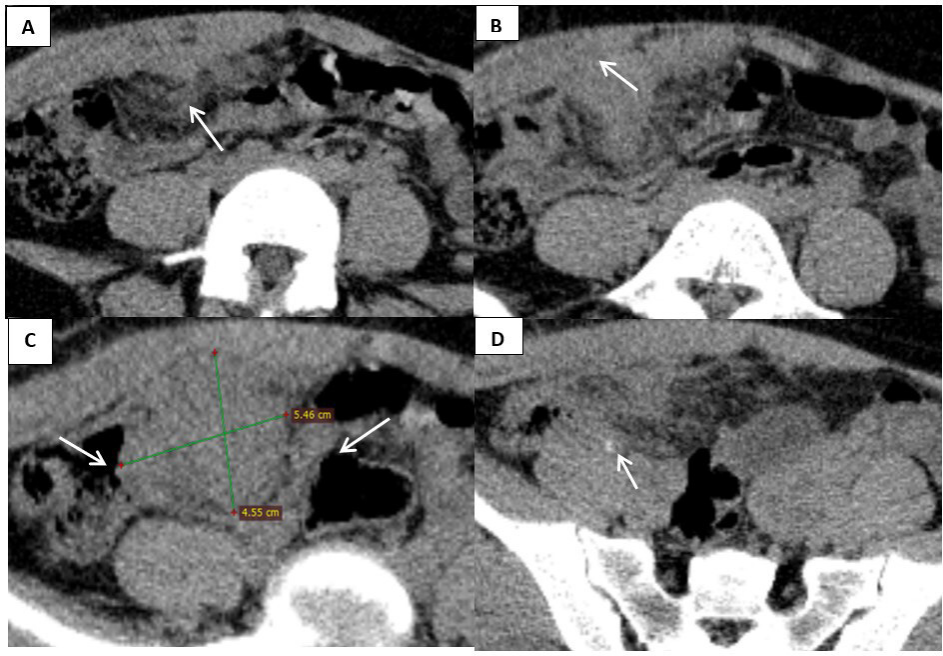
Non-contrast enhanced CT abdomen, showing a tumefactive soft tissue within the right iliac fossa measuring 4.5 × 5.5 cm in maximum axial dimensions, seen inseparable from the anterior abdominal wall (arrow, B), insinuated between the bowel loops (arrow, C), showing hyperdense focus (arrow, D), with related fat stranding (arrow, A).
